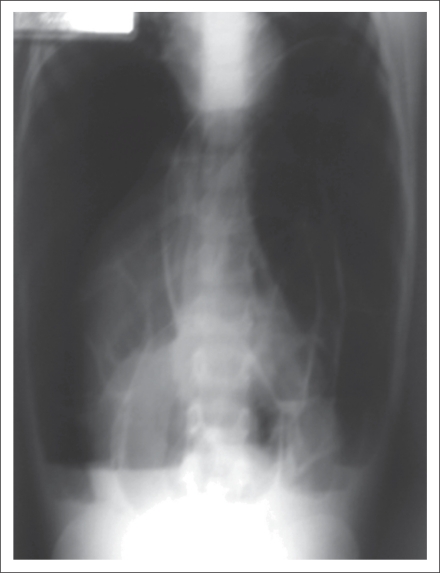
Plain abdominal roentgenogram showing dilated loops of bowel with extensive intramural gas
